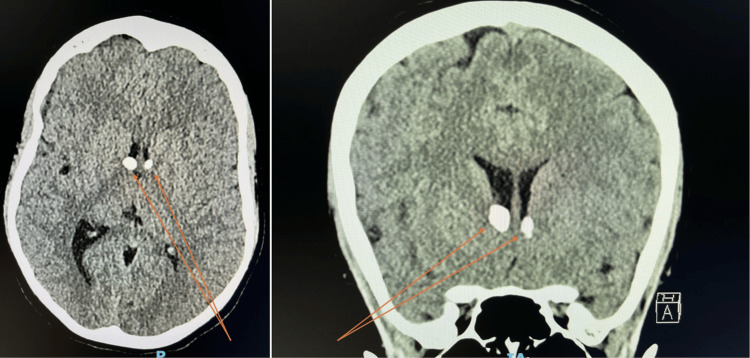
Follow-up CT imaging: axial and coronal views of the brain Arrows highlight calcified subependymal nodules within the lateral ventricles of the brain. These are recognised intracranial sequelae of TSC. Follow-up MRI imaging was requested for superior characterisation ahead of specialist neurological input.
